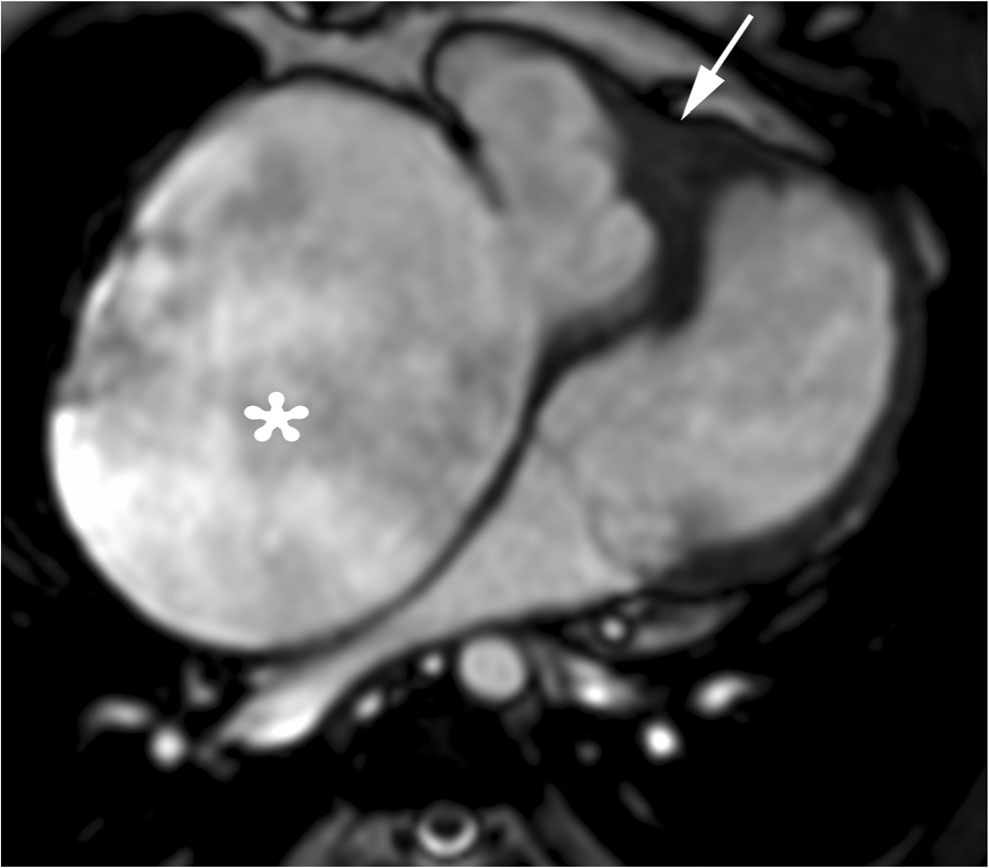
A 22-year-old woman with endomyocardial fibrosis. End-diastolic four-chamber cine SSFP MR image shows right ventricular apical thickening (*arrow*) and marked right atrial dilatation (*asterisk*) with signal void attributed to turbulent blood flow
